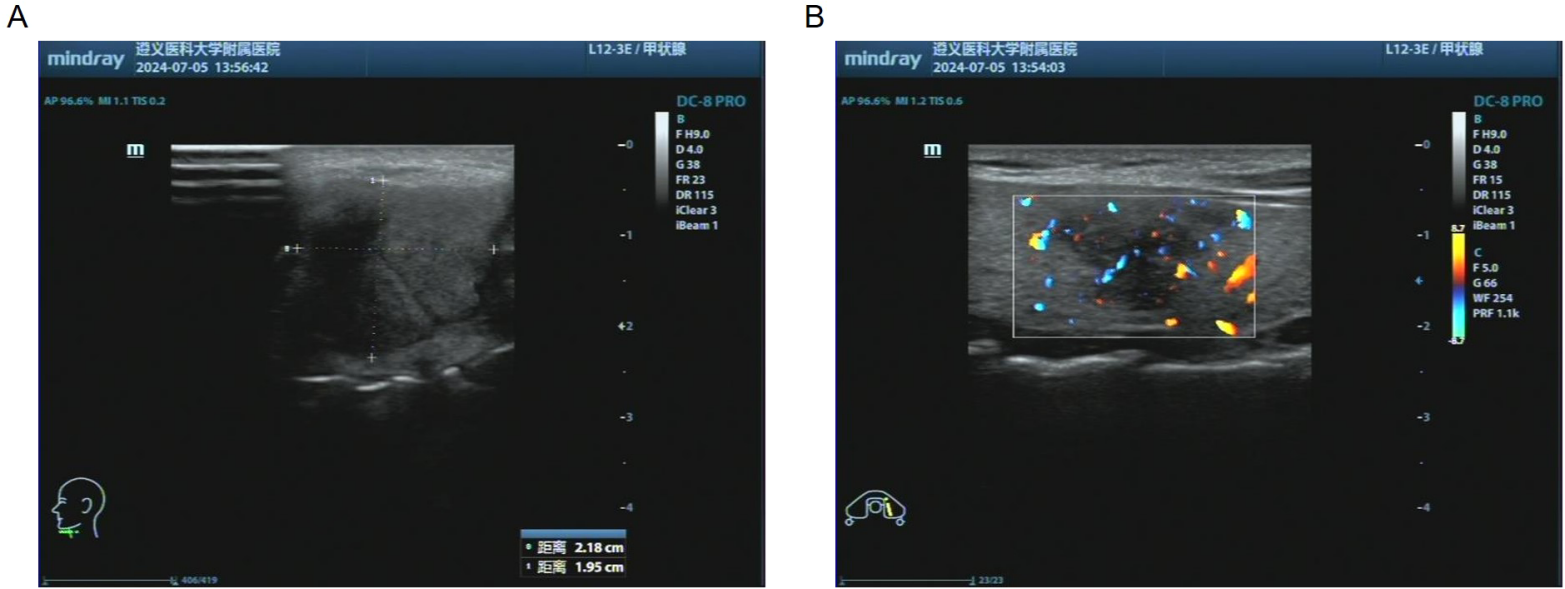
Results of preoperative cervical ultrasonography. (A) Ultrasonography revealed the hypoechoic area in the anterior cervical region, measuring 22×20 mm. (B) A 20×20 mm hypoechoic area of the left lobe thyroid was irregular in shape, ill-defined in boundary, and heterogeneous in internal echo.
